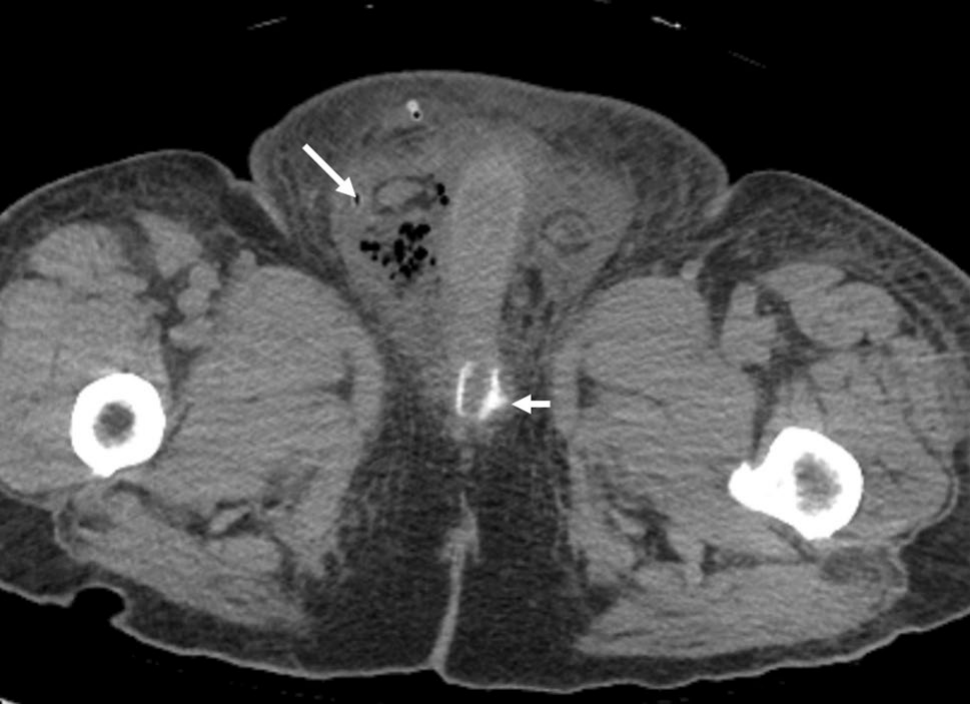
A 64 year-old presented with scrotal pain, redness and swelling. Axial CT shows the cuff around the bulbar urethra (short arrow). Multiple foci of gas in right hemiscrotum represented gas in an abscess (long arrow). The AUS was removed in its entirety and a Foley catheter placed for bladder drainage. VCUG done 2 months post-op (not shown) demonstrated complete urethral healing
